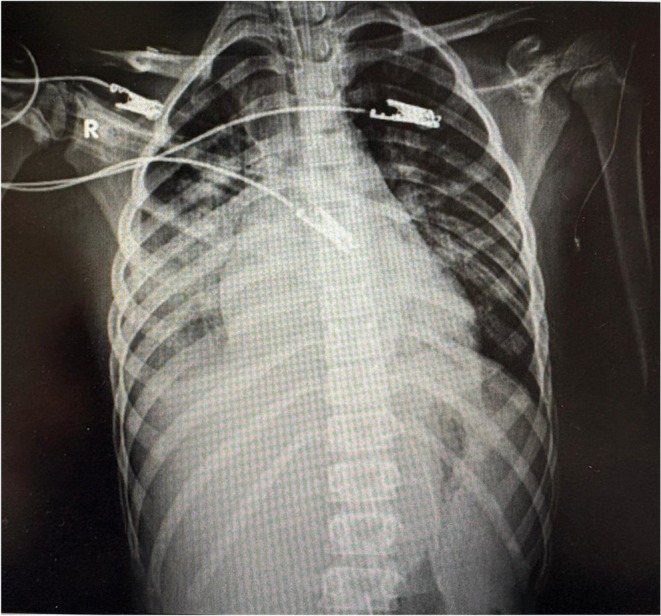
Follow‐up chest X‐ray after 2 days of mechanical ventilation showed an interval improvement of bilateral pulmonary infiltrates. Portable anteroposterior chest radiograph after 2 days of mechanical ventilation showed partial interval resolution of bilateral air‐space/interstitial opacities previously identified. Patchy opacities are predominantly in the right upper and mid‐lung zones, with mild residual left‐sided haziness. Findings are consistent with radiographic improvement of ARDS/acute respiratory failure with supportive ventilatory management.
